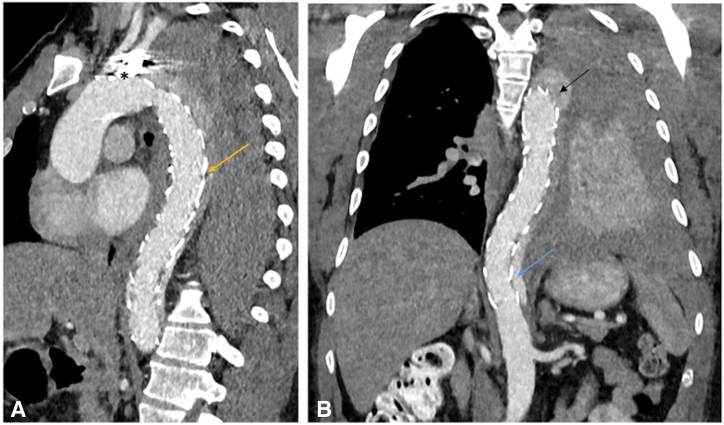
CT aortography after TEVAR. Sagittal oblique CT reformat (A) shows TEVAR stent in situ (*yellow arrow*) and the coiled left subclavian artery (*asterisk*). There is faint contrast opacification of the false lumen, which is better appreciated on the coronal oblique reformat (B), at the level of distal descending thoracic aorta (*blue arrow*) and distal arch (*black arrow*) with thrombosed false lumen in between, suspicious for endoleaks at proximal and distal ends of the stent. Residual hemothorax with atelectasis of the left lung is also noted. *CT*, Coronary angiography; *TEVAR*, thoracic endovascular aortic repair*.*
